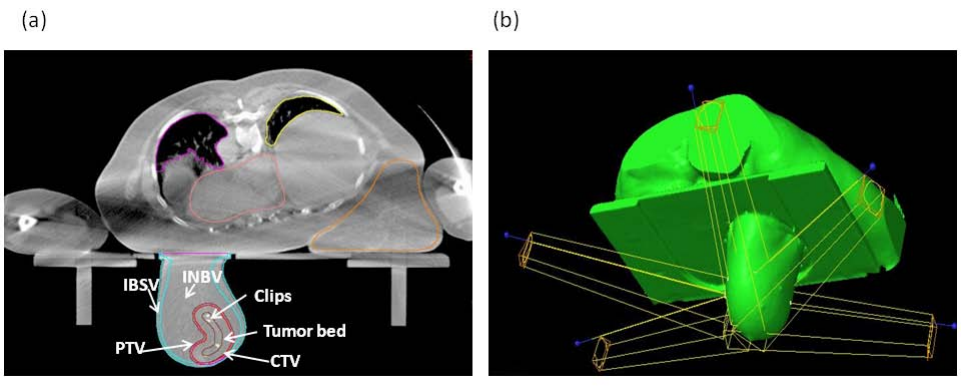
Contours and clips on CT slices. a) CT slice with clips, CTV, PTV and OARs, including INBV = ipsilateral normal breast volume, IBSV = ipsilateral breast skin volume. b) fields arrangement.
